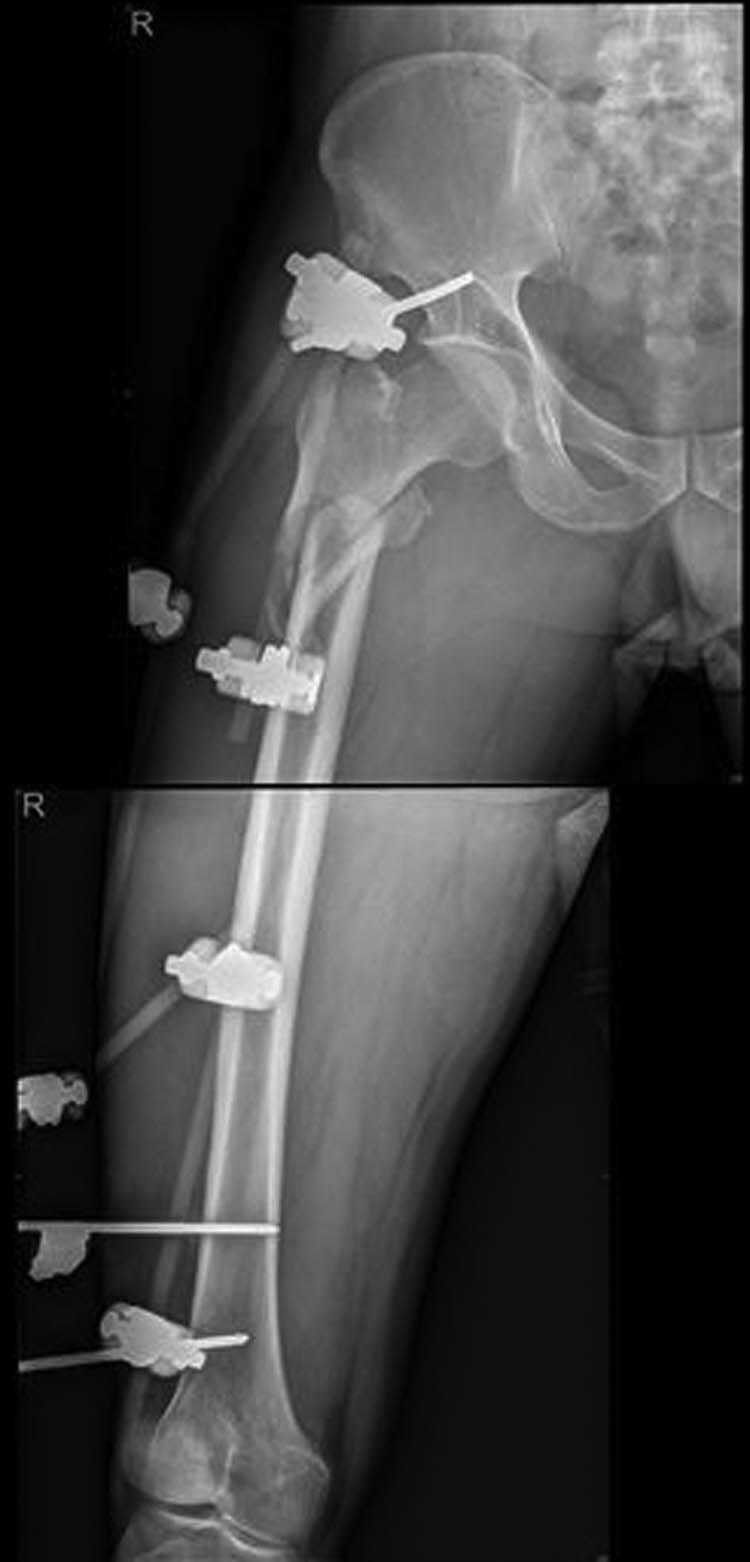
Postoperative X-ray showing good alignment following external fixation.
